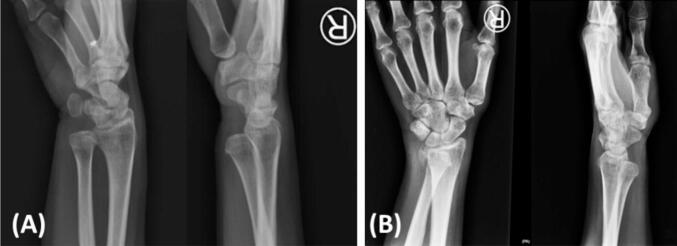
Plain radiograph after the initial injury (A) and plain radiograph at the outpatient clinic (B); both demonstrate a volar displacement of the ulna and a fracture of the ulnar styloid.
